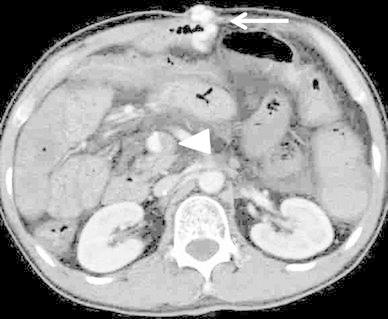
Contrast-enhanced CT scan reveals mesenteric varices (*arrow*) feeding the abdominal wall vein. All varices were directly connected. There is thrombosis in the mesenteric vein (*arrowhead*)
